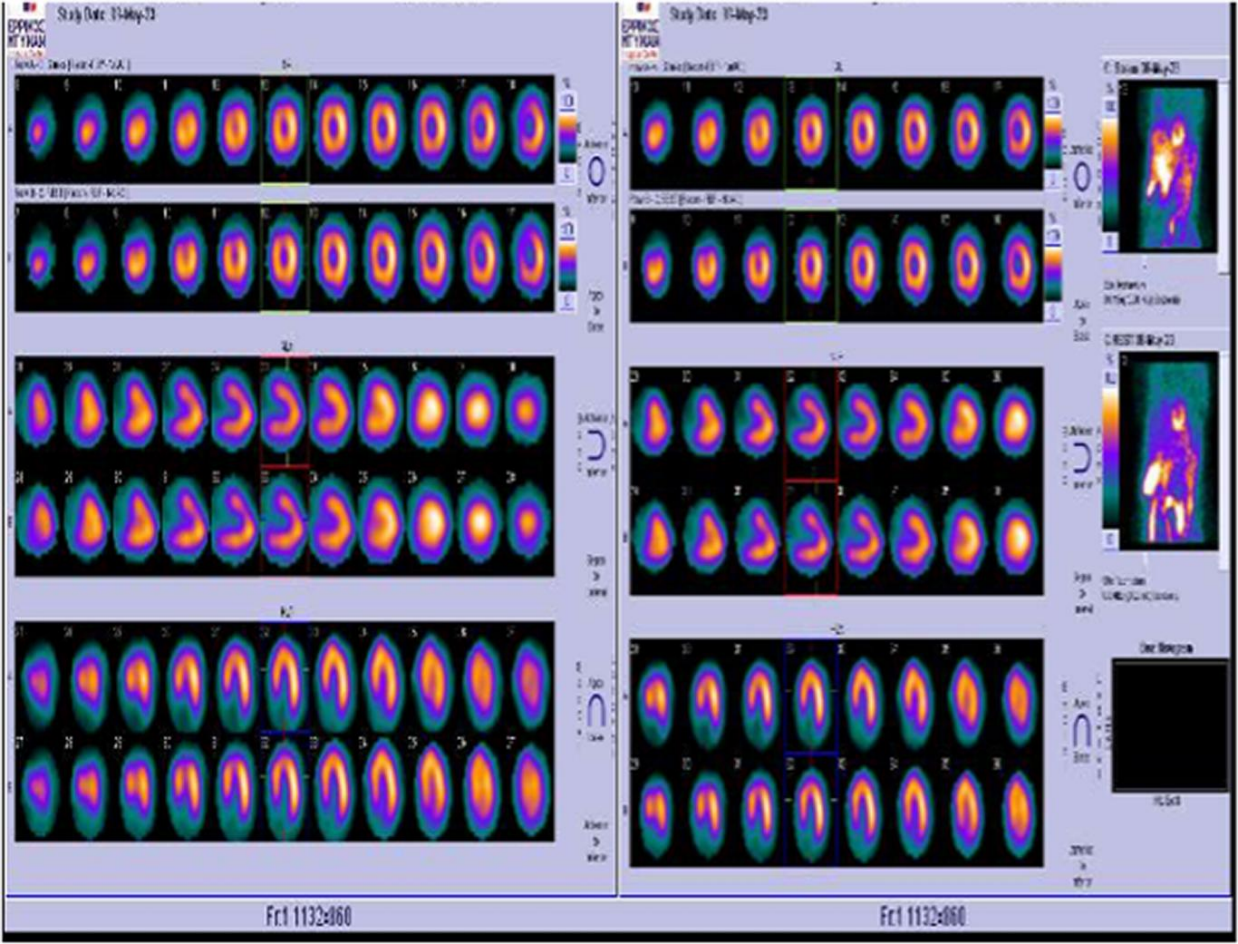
Single-photon emission computed tomography with exercise on treadmill post-revascularization.
